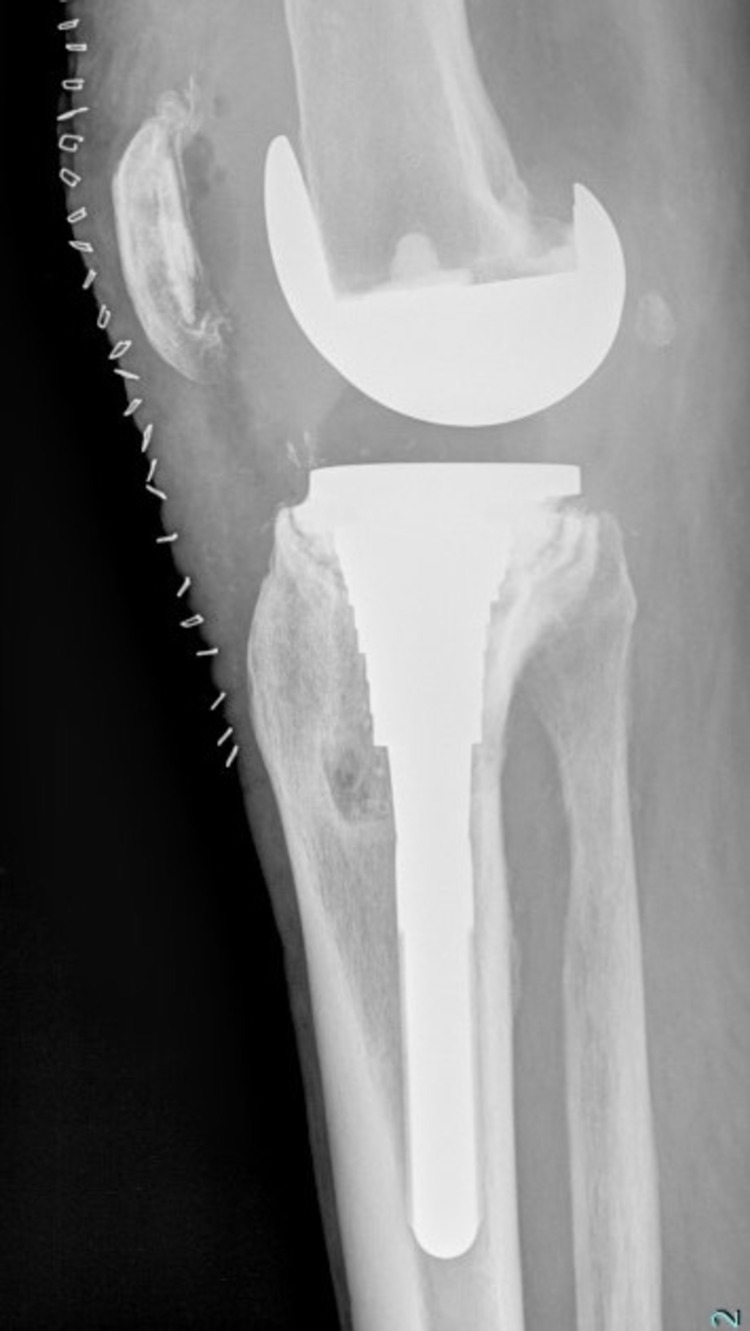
Early postoperative X-ray lateral view showing lack of metal-bone contact in the anterior and posterior aspect of the metaphyseal sleeve.
